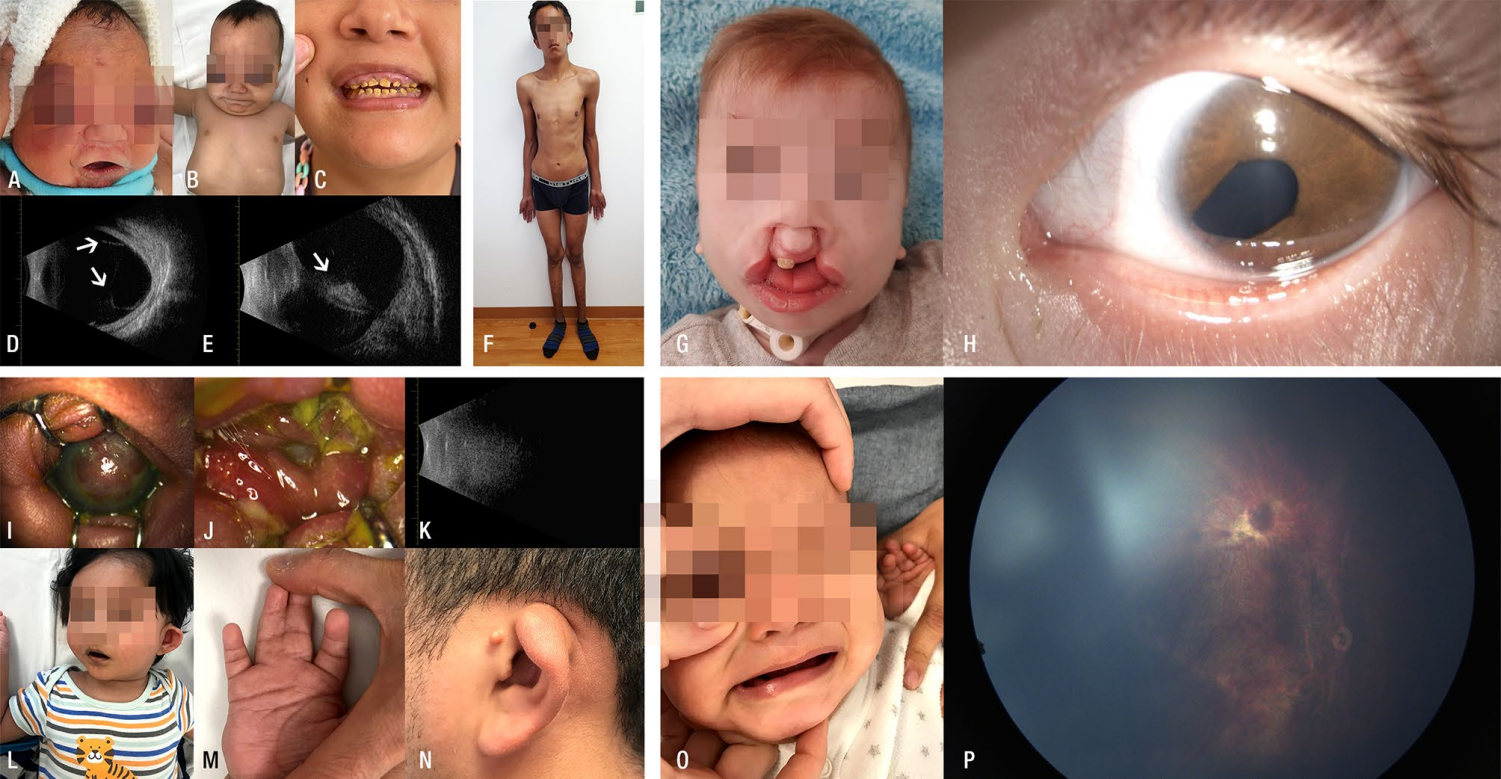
Clinical features of the five patients with clinically relevant variants identified by WES. (A–E) Patient with JLSM syndrome exhibits bilateral buphthalmos, palpebral edema and ecchymosis (A, B); amelogenesis in the proband´s sister (C); b-mode ocular ultrasound with axial length of 24.17 mm and anterior posterior vitreous detachment (D) followed by retinal detachment in the OD (E). (F) Patient with Malan syndrome who presented dolichostenomelia, pectus excavatum, and a prominent forehead. (G,H) Patient with DEDDFA displays features of medial cleft lip and palate and iris coloboma. (I–N) Patient with LADD1 syndrome with corneal opacity in the OD (I) and clinical anophthalmos in the OS (J). B-mode ocular ultrasound revealed no ocular structures (K), facial dysmorphism (L), syndactyly (M), and abnormal pinna morphology in the affected father (N). (O,P) Fundoscopy revealed optic nerve aplasia in OS in a patient with PAPRS.
